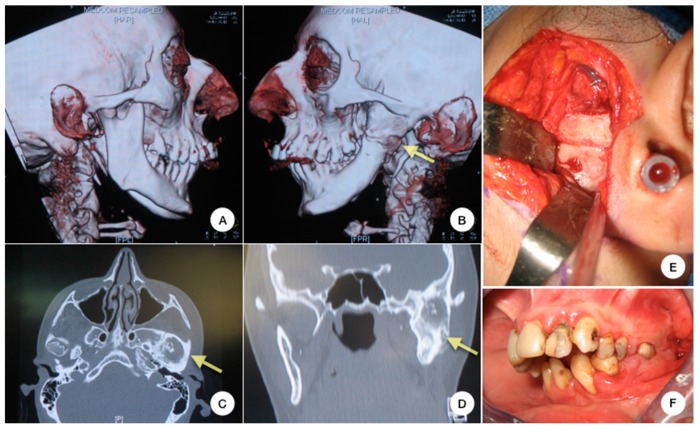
Invasive treatment of a patient suffering from TMJ (temporomandibular joint) ankylosis: Right lateral view of a 3D CT scan reconstruction of the head: the right TMJ is affected by joint space narrowing (A) and the left TMJ space has completely disappeared and been replaced by an osseous block (B). This replacement of the left TMJ by an osseous block of ankylosis is seen on transversal (C), and on coronal (D) CT scan sections. Intraoperative view of invasive treatment: the osseous bloc of ankylosis replacing the left TMJ space is approached through a pre-auricular incision (E). Preoperative intraoral photograph showing the absence of mouth opening (F).
